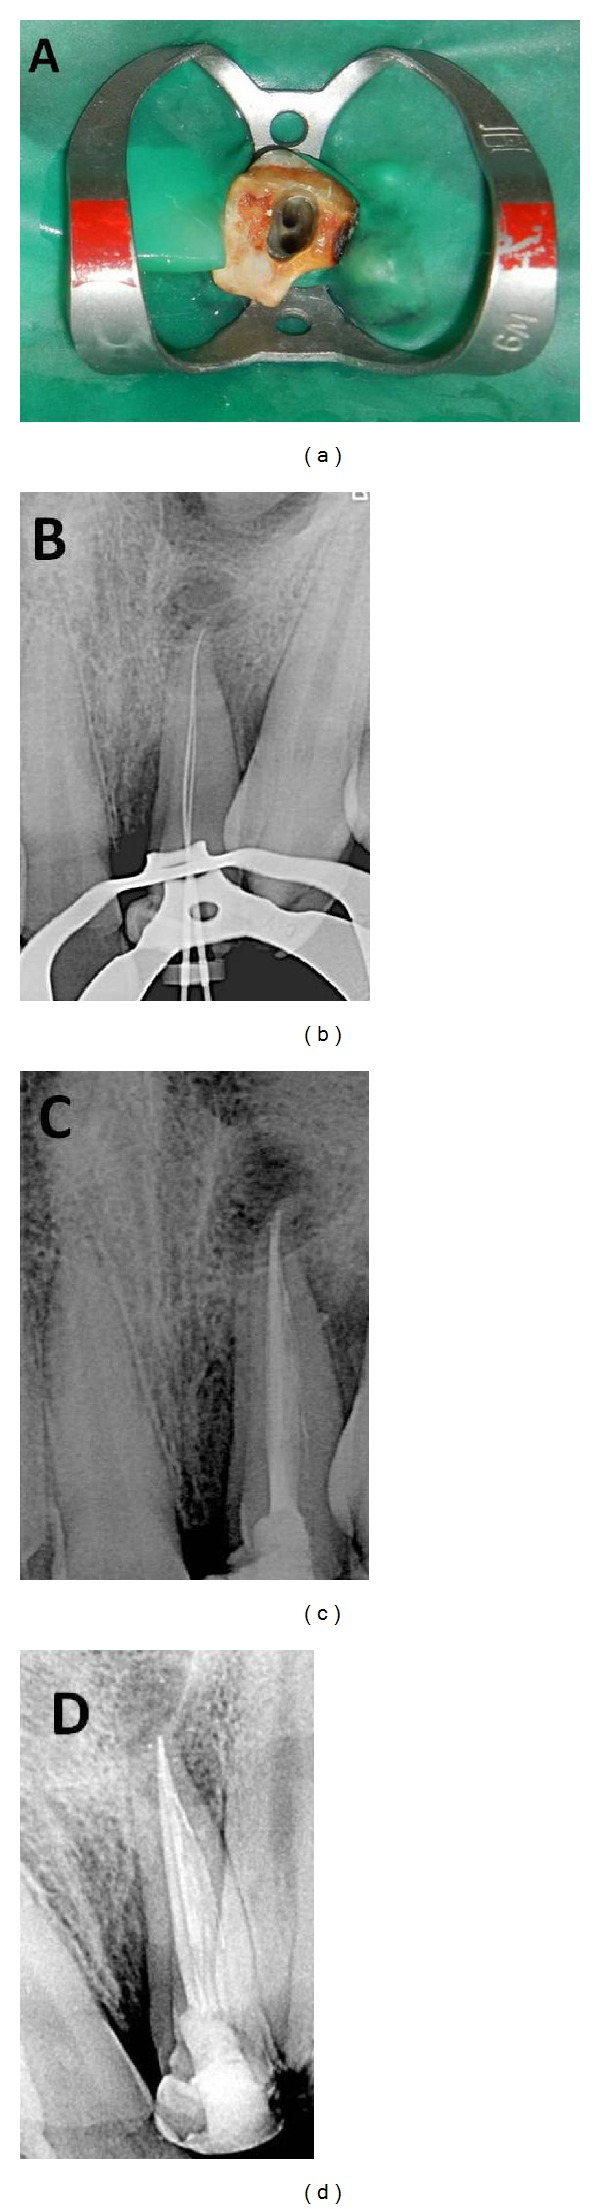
(a) Clinical picture showing two distinct canal openings: one labial and one palatal in the central incisor. (b) Radiographic determination of working length. (c) Postoperative periapical radiograph demonstrating completed nonsurgical root canal therapy. (d) Postoperative periapical radiograph with angulated projection.
